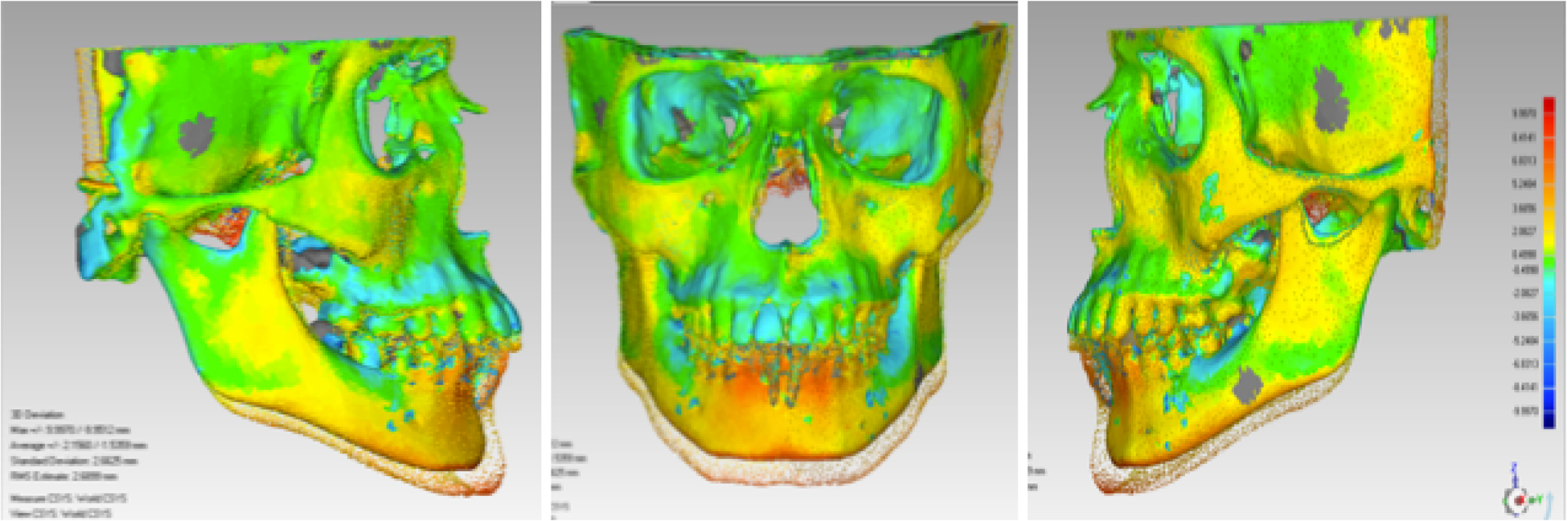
Print screen of the mandible and the maxilla’s superimposition on the cranial base using the software Geomatic Qualify 2013To evaluate gingival recession in the lower incisor area, comparison between dental casts and photographs from the beginning and the end of the Herbst appliance phase will be done. The presence or absence of gingival recession for each lower incisor will be noted for each lower incisor for each time point. In the presence of recession, a ‘yes’ will be marked. The photographs will be used to confirm [18]To evaluate root resorption, comparison between CBCT from the beginning and the end of the Herbst phase will be used for each lower incisorTo explore young people’s experience of using the two types of Herbst appliance, the following methods will be used:4.1.Self-reported questionnaire about patient discomfort4.2.Semi-structured interviews
